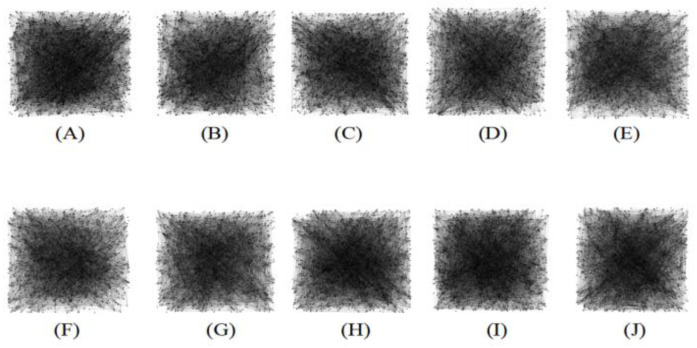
Corresponding graphs generated based on pixel-level graph construction. (A–E) represent head CT images with motion artifacts. (F–J) represent head CT images without artifacts.
